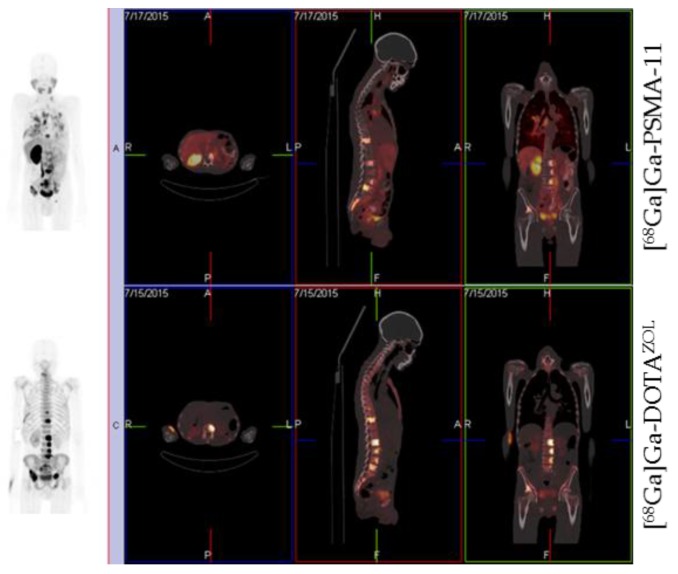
Whole-body PET/CT 60 min p.i. 170 MBq [^68^Ga]Ga-PSMA-11 and 155 MBq [^68^Ga]Ga-DOTA^ZOL^, respectively, in a prostate cancer patient (71 years old, Gleason 4 + 4).
